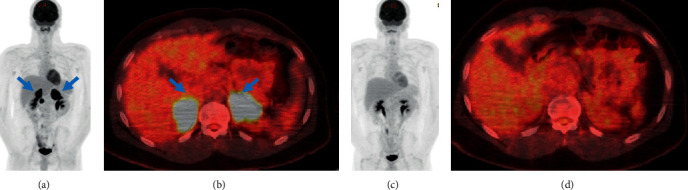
Pretreatment coronal maximum-intensity-projection (a) and axial fused FDG-PET (b) images show enlarged and hypermetabolic bilateral adrenal glands (blue arrows) with SUV max of 17.1 and 14.1 on the right and left respectively. Posttreatment coronal maximum-intensity-projection (c) and fused axial FDG-PET (d) images show resolution of previously seen hypermetabolic activity of bilateral adrenal glands two months following CAR-T.
